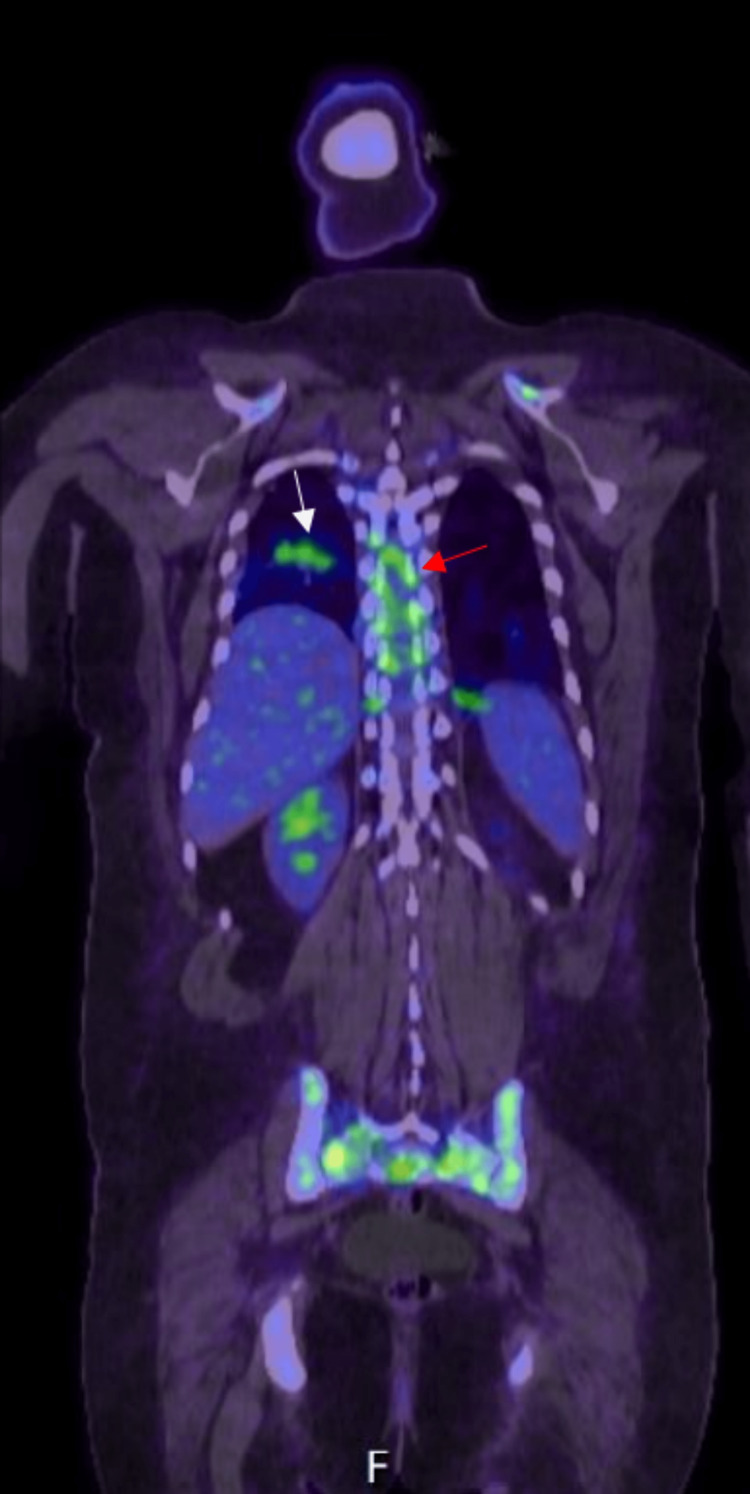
Coronal fused PET-CT skull base to mid-thigh. The PET scan revealed multiple hypermetabolic pulmonary masses (white arrow) compatible with lymphoma and multiple hypermetabolic osseous lesions compatible with malignant involvement, including multiple vertebrae (red arrow), sacrum, and pelvis.
